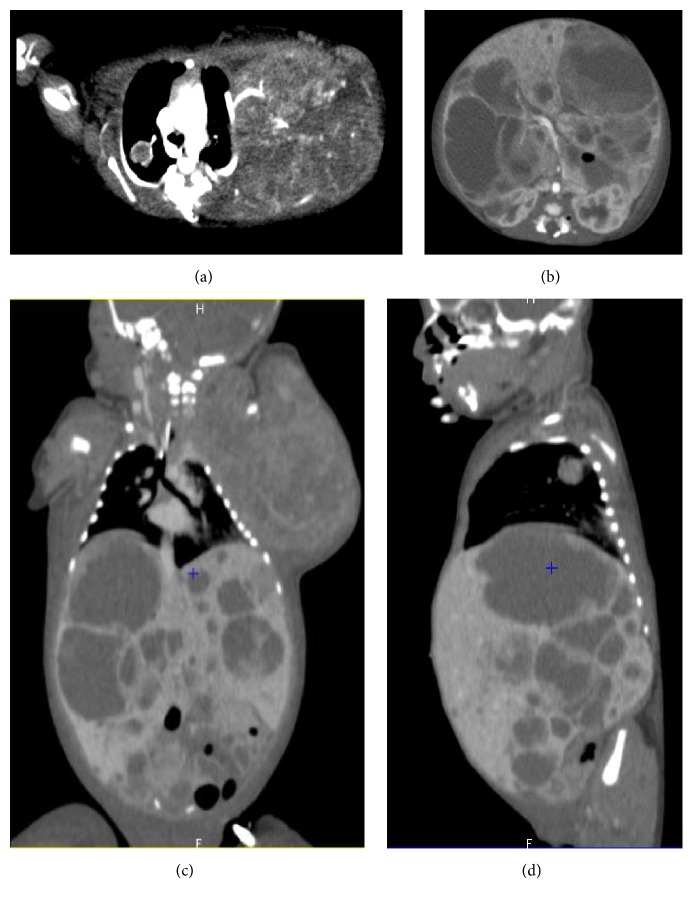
CT scan with a contrast agent showing the axillary mass compressing the chest (a) and numerous intra-abdominal tumors involving the liver, spleen, and right kidney (b, c, d) in axial, frontal, and sagittal views.
